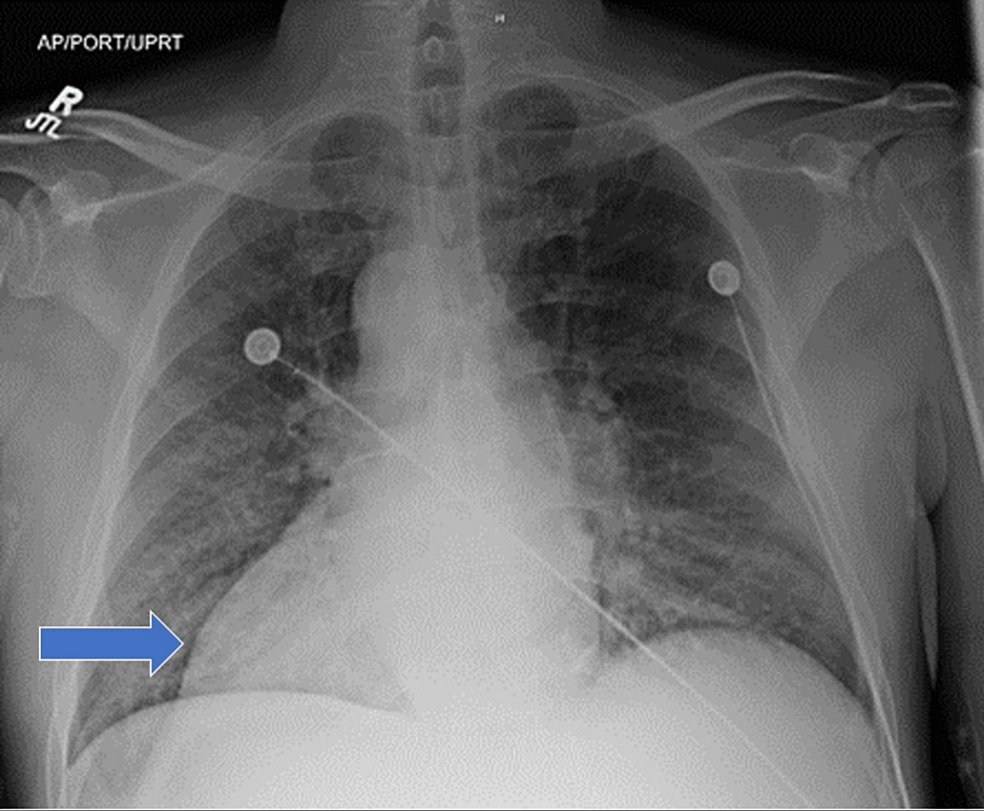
Chest x-ray AP view showed a cardiac malposition, dextroversion, in which the apex of the heart is pointing to the right (blue arrow)
